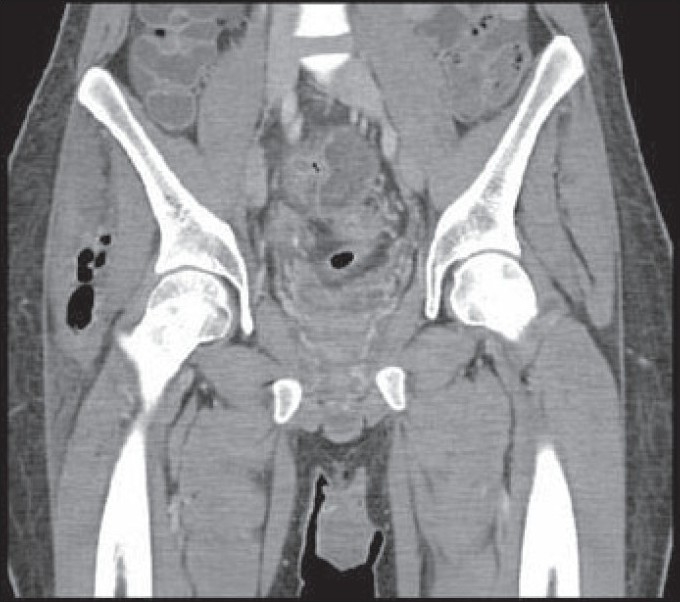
CT scans of the abdomen and pelvis coronal section
